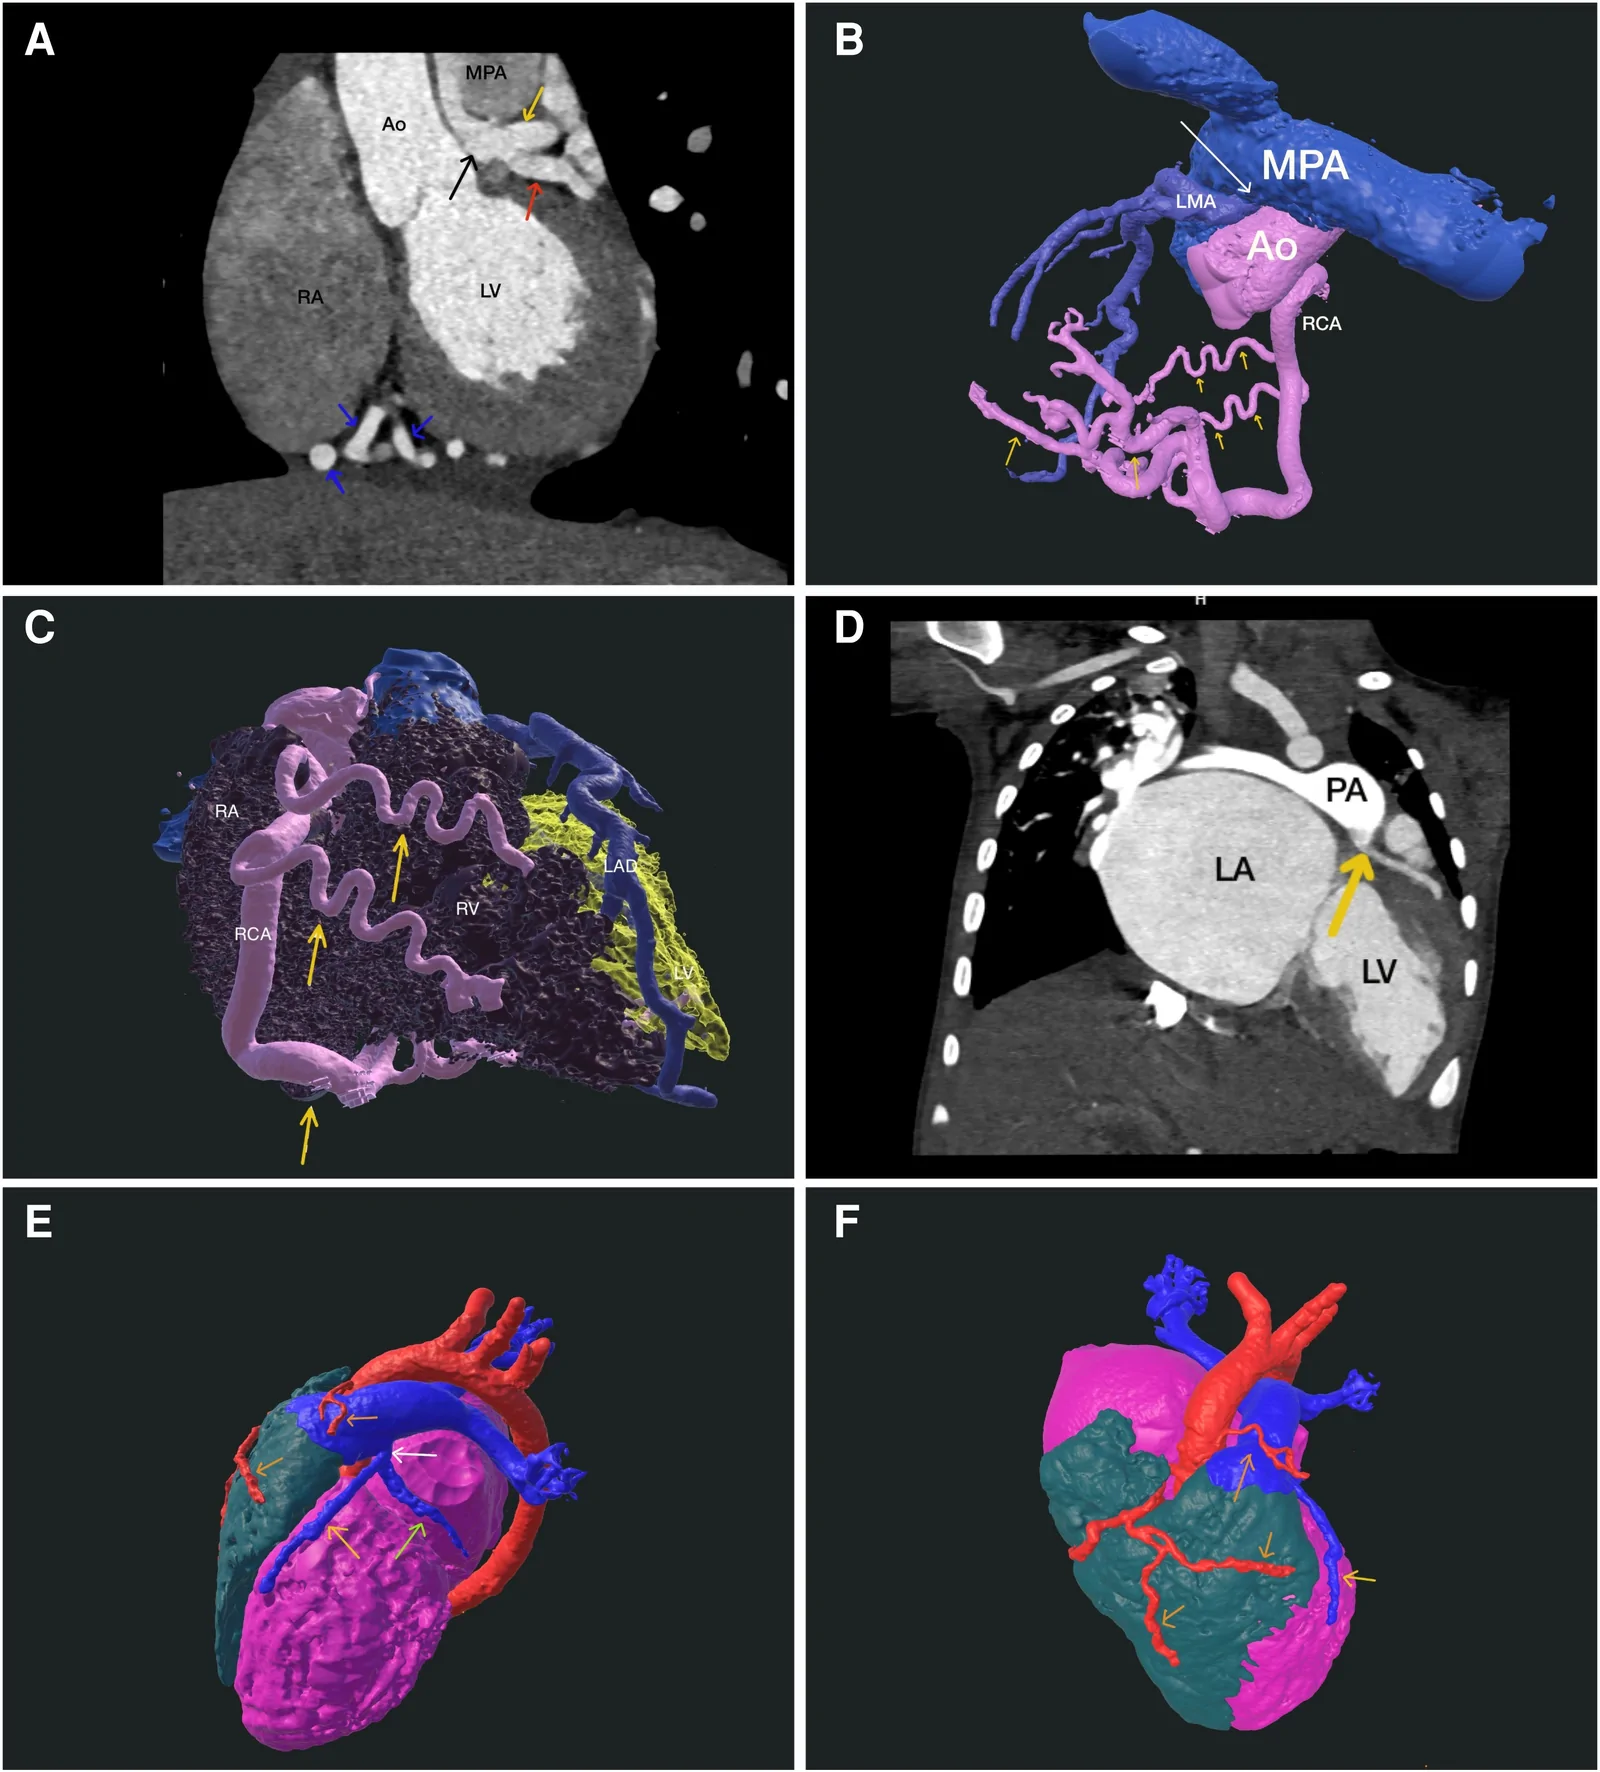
ALCAPA in 3D: from pulmonary origin to epicardial collateral network. A 13-year-old girl who presented with palpitations. (*A*) Computed tomography demonstrated anomalous left coronary artery from the pulmonary artery, with the left main coronary artery (LMA; black arrow), left anterior descending artery (LAD; yellow arrow), left circumflex artery (LCx; red arrow), and collateral vessels (blue arrows) identified. Ao: aorta MPA: main pulmonary artery RA: right atrium LV: left ventricle (*B*) A posterior view of the virtual reality rendering demonstrates the dilated left coronary arteries arising from the MPA (white arrow) and multiple collaterals (yellow arrows) from the right coronary artery (yellow arrows). Aorta, RCA and its branches: pink; Pulmonary arteries, LMA and its branches: blue (*C*) A second virtual reality view demonstrates the extensive epicardial collateral network (yellow arrows) RV: transparent purple; LV: transparent yellow. (*D*) An 11 month old infant who presented with failure to thrive. CT showed the origin of the LMA (yellow arrow) from the main pulmonary artery (PA) and a markedly dilated left atrium (LA) (*E*,*F*) Virtual reality images provide enhanced depth perception and spatial definition, demonstrating the LMA(white arrow), LAD(yellow arrow) and LCx (green arrow) as well as collateral vessels from the RCA(orange arrows). Pulmonary arteries, LMA, LCx, LAD: blue; aorta, RCA and branches: red; right ventricle: green, left ventricle and left atrium: pink.
